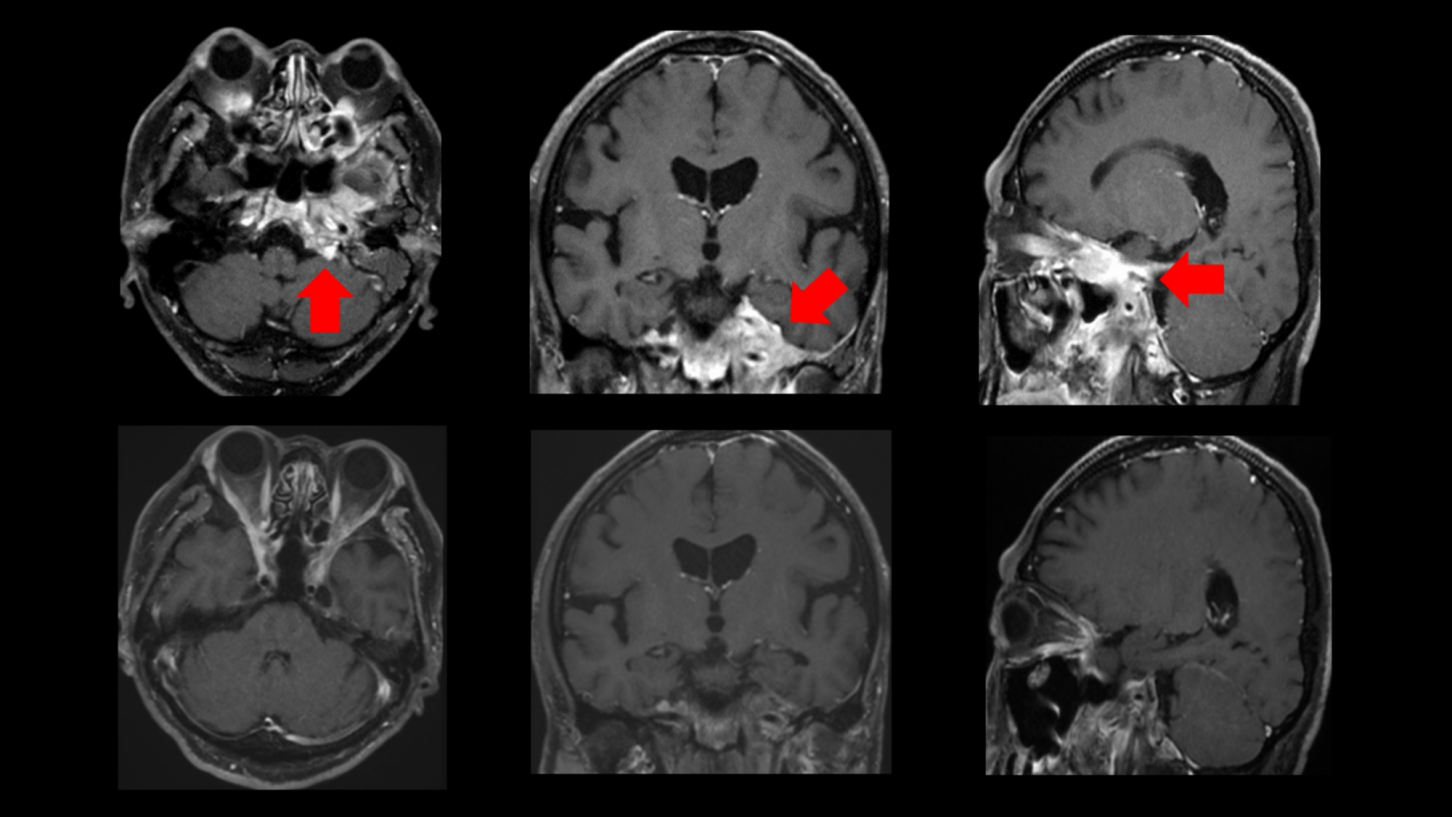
Contrast-enhanced MRI of Case 1 Contrast-enhanced MRI before CyberKnife (top) and after CyberKnife (bottom). The tumor in the petroclivus (red arrow) disappeared after treatment. MRI: magnetic resonance imaging
